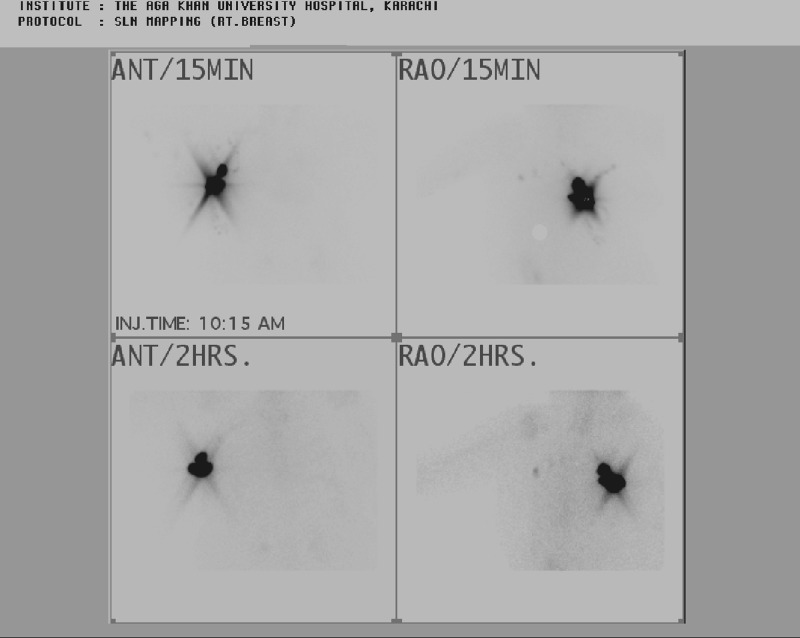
Radionuclide sentinel node mapping and scintigraphy Fifteen-min images show dense tracer uptake over the site of injections and few ill defined areas of abnormal uptake are seen in the right axilla. One hour images redemonstrated dense tracer uptake over the sites of injection and a well outlined and an ill defined area of abnormal tracer uptake in the right axilla. Two nodes were marked over the skin with the help of a hot marker under the gamma camera.
